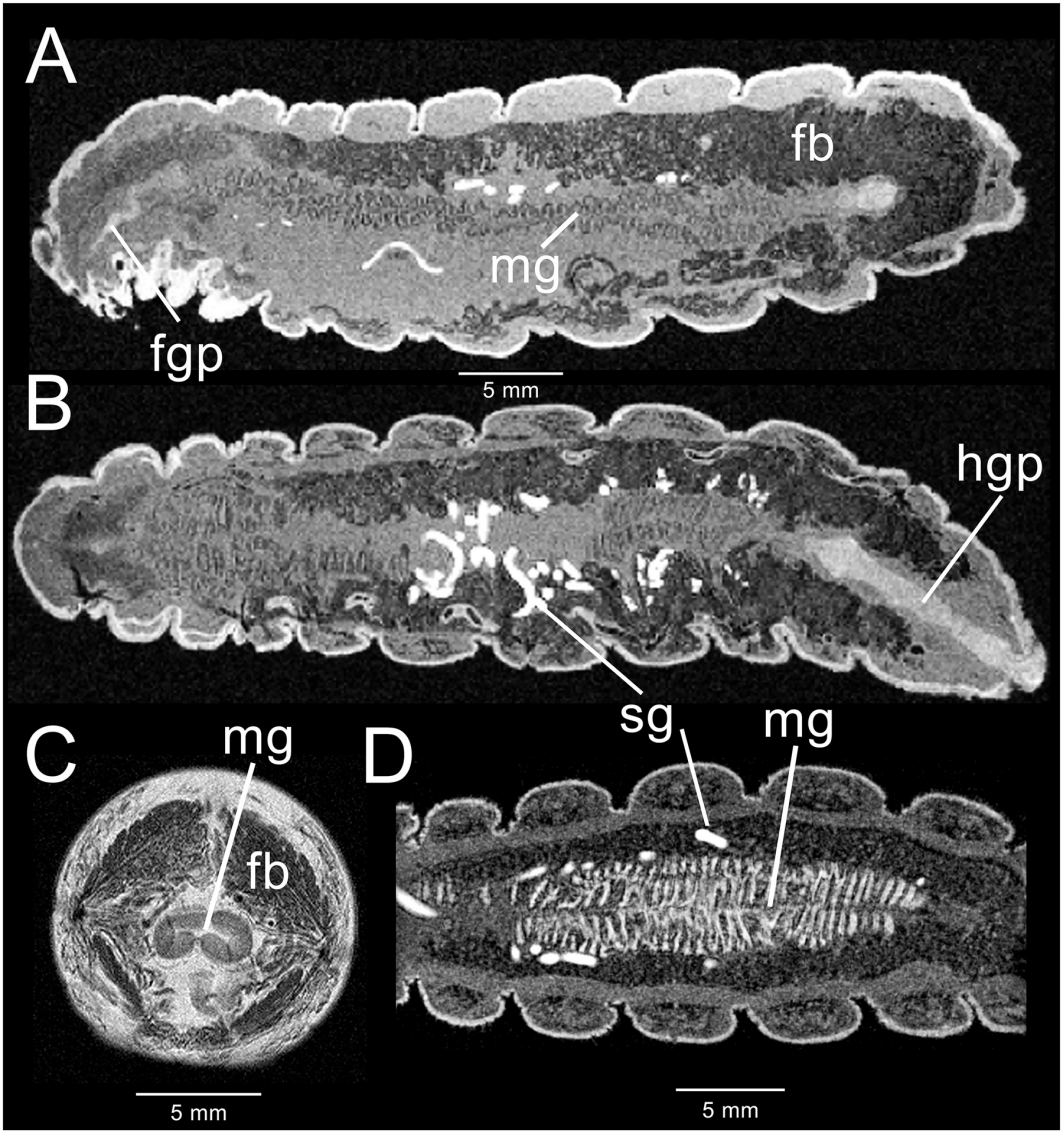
MR imaging data acquired from two different 180 hour fifth instar *M*. *sexta*. The insect shown in A-C was not previously fed CA; the insect shown in D was previously fed CA. (A) 3D-GRE mid-sagittal section displaying the fat body (fb), foregut “plug” (fgp) and highly shrunken midgut. (B) Coronal section of the same insect, revealing the silk glands (sg) and hindgut “plug” (hgp). (C) Transverse 2D-SE slice of the same insect through the second abdominal segment, showing collapse of the midgut. (D) 3D-GRE coronal section through abdominal segments 3–6, displaying the collapsed midgut and the silk glands.
